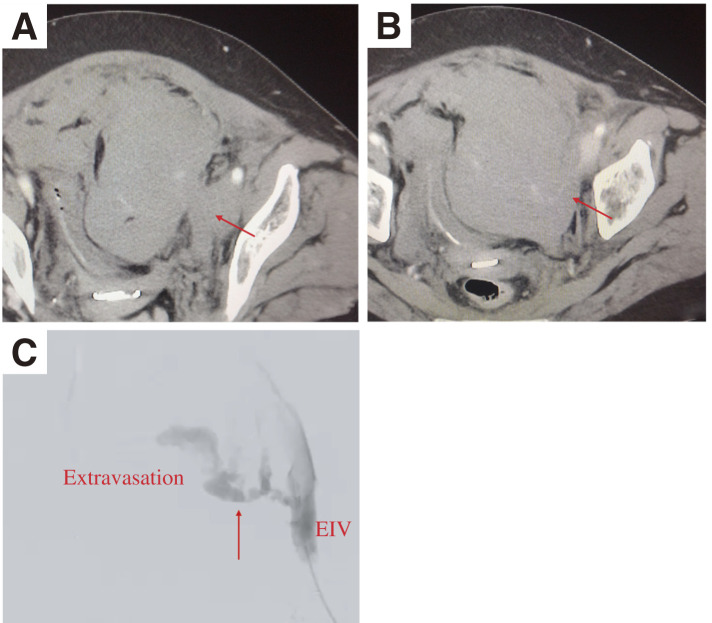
Preoperative and initial intraoperative diagnostic findings. (A) Preoperative contrast-enhanced CT showing the common iliac vein suspected to be obstructed (arrow). (B) CT image revealing extravasation continuous from the external iliac vein (arrow). (C) Initial intraoperative venography confirming extravasation from the external iliac vein (arrow) and the absence of the common and internal iliac veins due to obstruction. EIV, external iliac vein; CT, computed tomography
